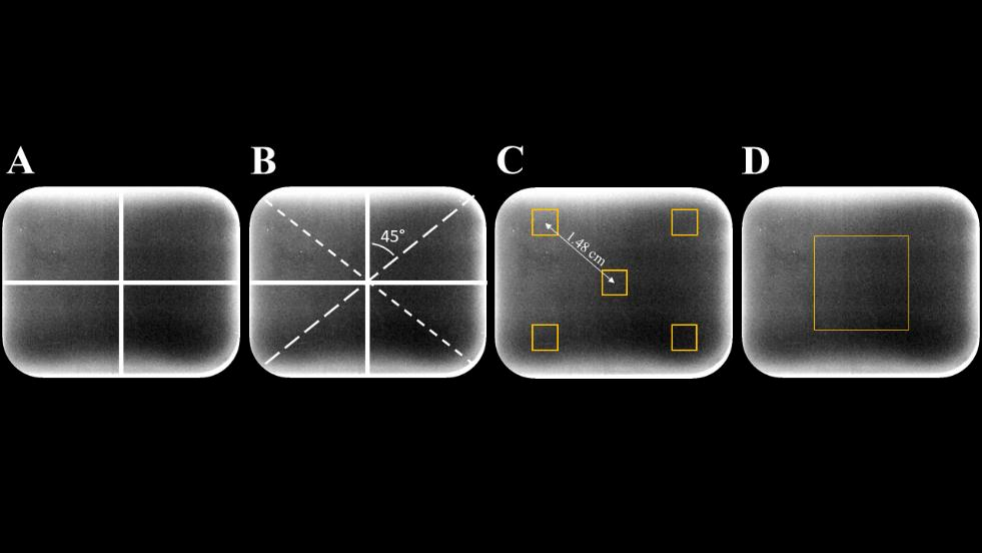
Objective assessment of image quality. (A) - Two lines were determined: one equally dividing the radiographic image into superior and inferior; and a line perpendicular to the first one, equally dividing the image into left and right sides. (B) - Two bisecting lines (45°) were drawn in the crossing area of the first two lines. (C) - Five square ROIs of the same size (4 x 4 mm) were drawn in distinct areas of the image (one ROI in the center of the image, and four ROIs on the bisector lines) to measure image noise. The distance between the center of the central ROI and the center of the other ROIs was standardized in 1.48 cm. (D) - A larger square ROI (1.48 x 1.48 cm) covering the central area of the image was determined to measure the was determined to measure the mean (image brightness) and the standard deviation (image uniformity) of gray values.
